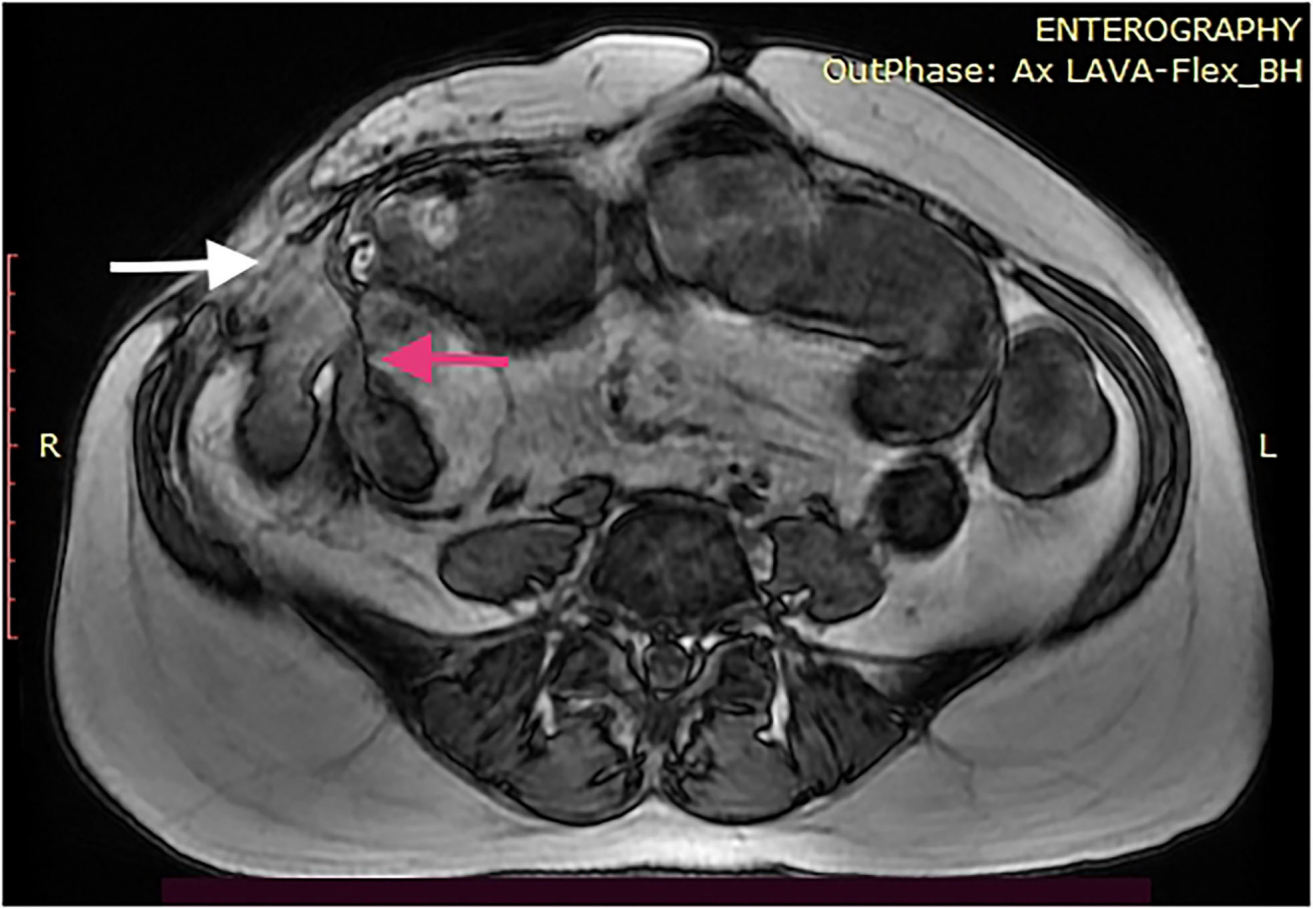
MRI enterography of a 46-year-old woman with CD with an enterocutaneous fistula (ECF) at the right iliac fossa (white arrow). She has undergone two ileocolic resections previously and a stricturoplasty of the end to end anastomosis within a 7-year period. The image demonstrates proximal bowel dilatation and a possible distal stricture (red arrow).
